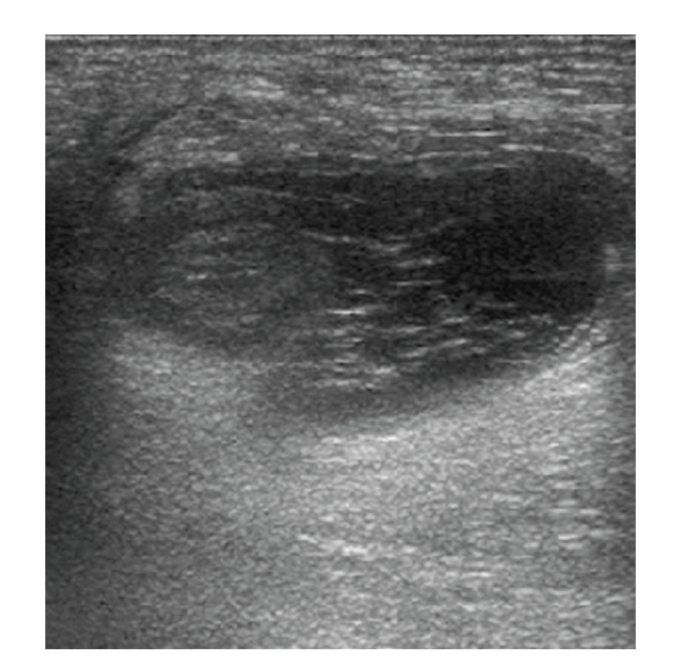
Preoperative ultrasound scan of right groin swelling showing a presumed indirect inguinal hernia containing one bowel loop and fatty omentum.
